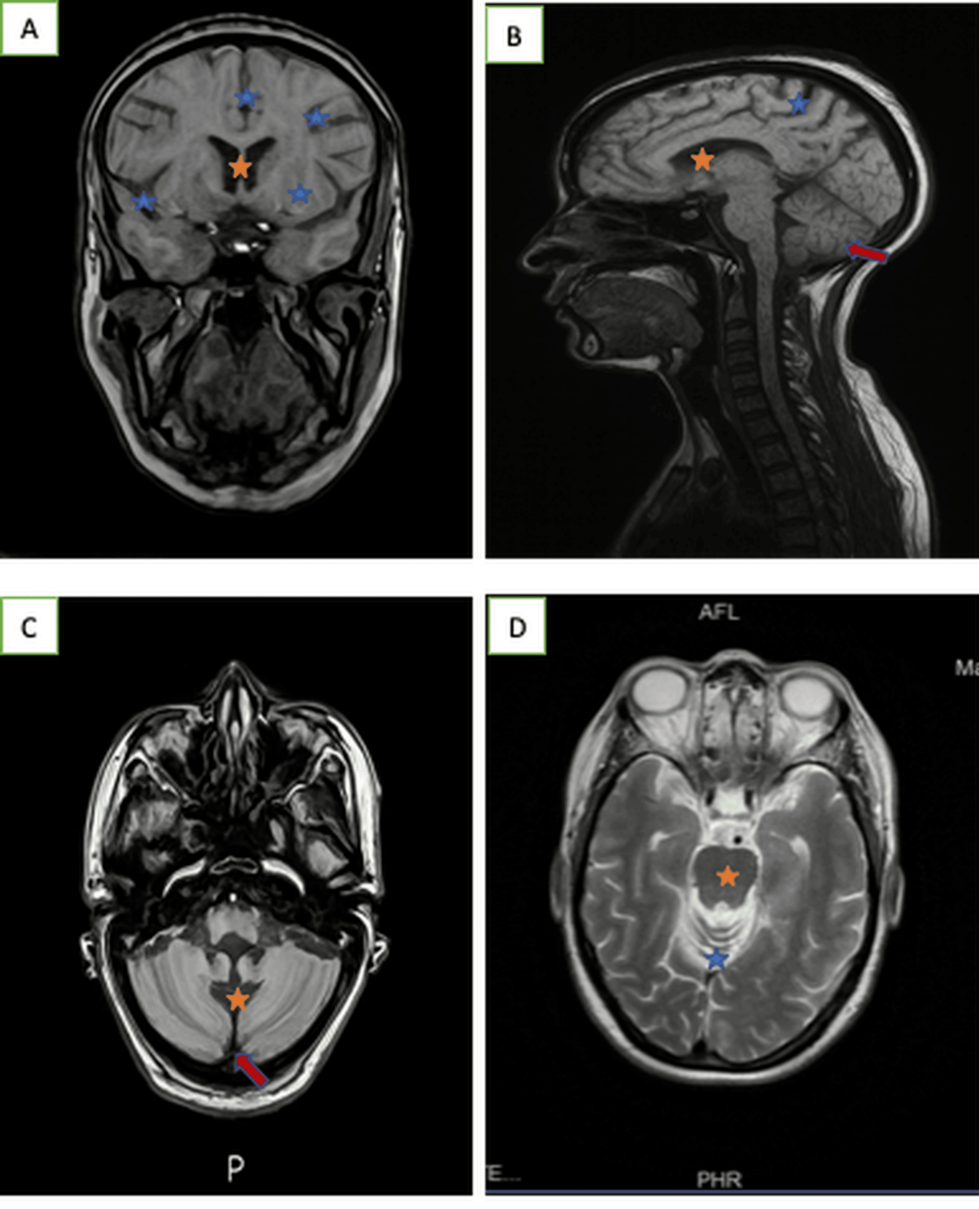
Brain MRI in different planes ((A) coronal cerebral T1-weighted sequence, without contrast, (B) median sagittal T1-weighted sequence, (C) axial T1-weighted sequence, (D) axial T2-weighted sequence) showing enlargement of the cerebellar sulci (blue stars) and folia with dilatation of the fourth ventricle (orange stars), consistent with diffuse cerebellar atrophy (red arrows) in the context of paraneoplastic cerebellar degeneration. MRI: magnetic resonance imaging; AFL: anterior funicular lesion; PHR: posterior horn region
